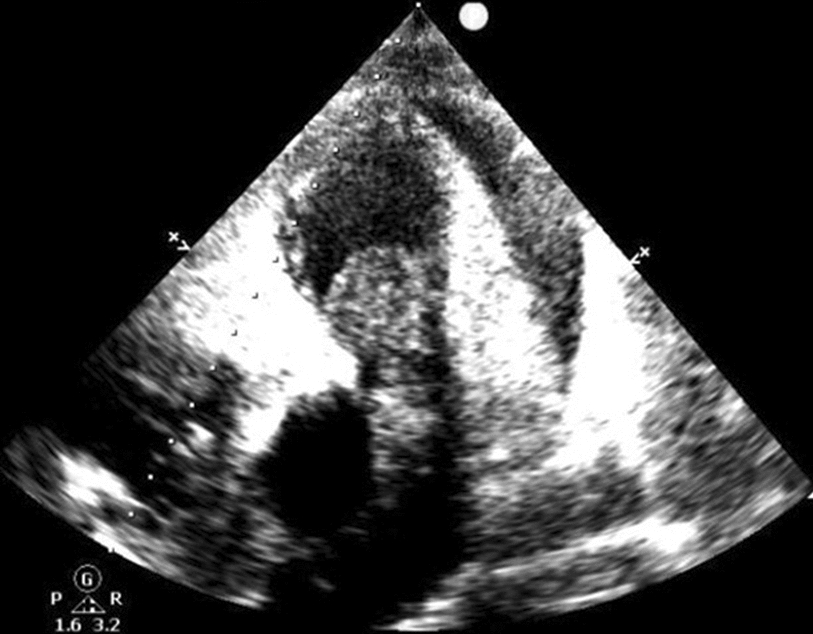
Long axis of pulmonary artery of transthoracic echocardiography. Arrow indicates the location of the lesion
